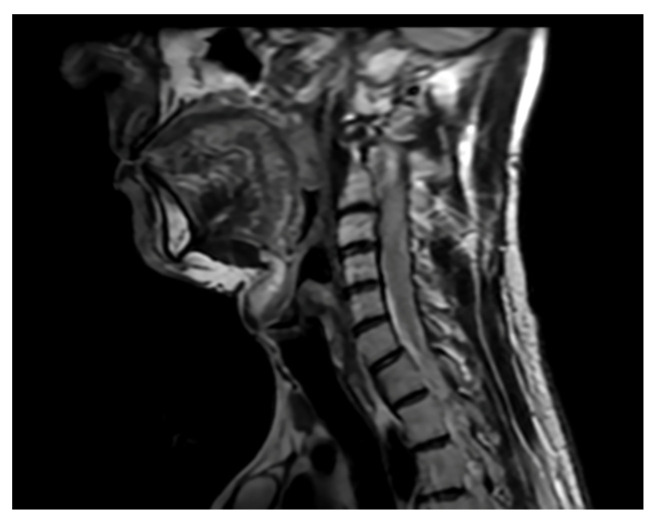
Single sagittal scan of the cervical spine. Follow-up MRI 2 months postoperatively. MRI T2.
